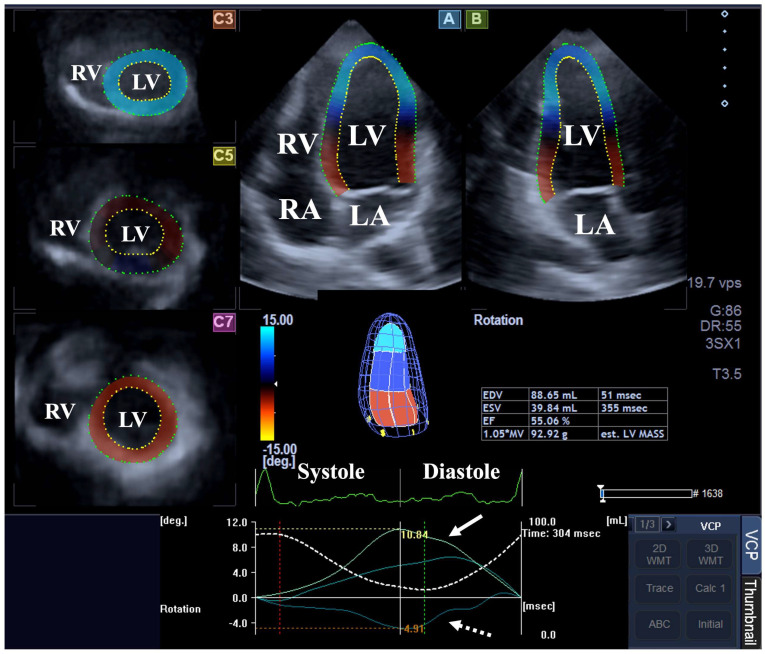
Assessment of the left ventricular (LV) rotational parameters by three-dimensional (3D) speckle-tracking echocardiography. Apical long-axis four-chamber (A) and two-chamber (B) views and short-axis views at the basal (C3), midventricular (C5) and apical LV levels (C7) are shown with a virtual 3D model of the LV and calculated LV volumetric data. Apical (white arrow) and basal (white dashed arrow) time—LV rotational curves are presented together with a curve representing time—LV volume changes (dashed white line) during the heart cycle. Abbreviations: LA = left atrium; LV = left ventricle; RA = right atrium; RV = right ventricle; EDV = end-diastolic volume; ESV = end-systolic volume; EF = ejection fraction.
